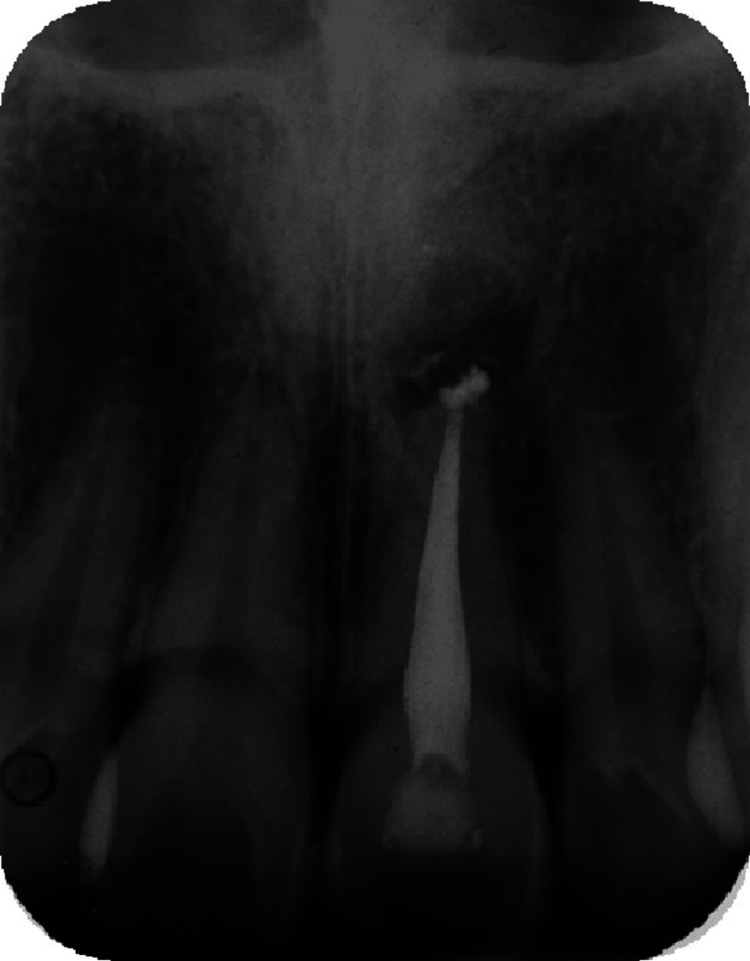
Preoperative radiograph of tooth 21
